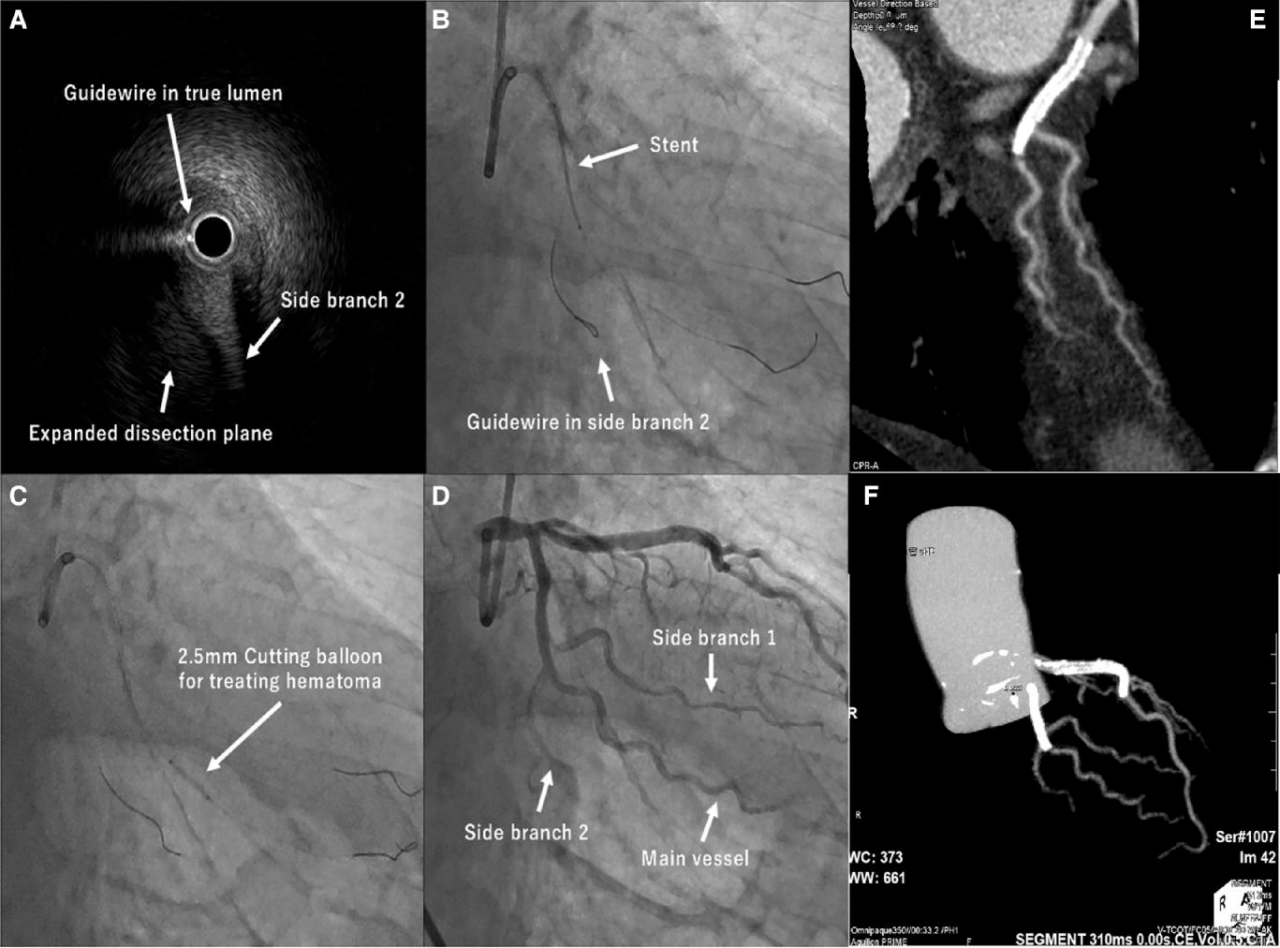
Images after the successful imaging-based antegrade dissection and re-entry. *(A)* The guidewire course was intraplaque and the side branch 2 originated from the same lumen. *(B)* After inserting a protection guidewire into side branch 2, we placed a 2.5/28 mm stent. *(C)* Cutting balloon was used to create re-entries for the huge distal site haematoma created by STAR. *(D)* Final angiography. *(E* and *F)* Follow-up coronary CT.
